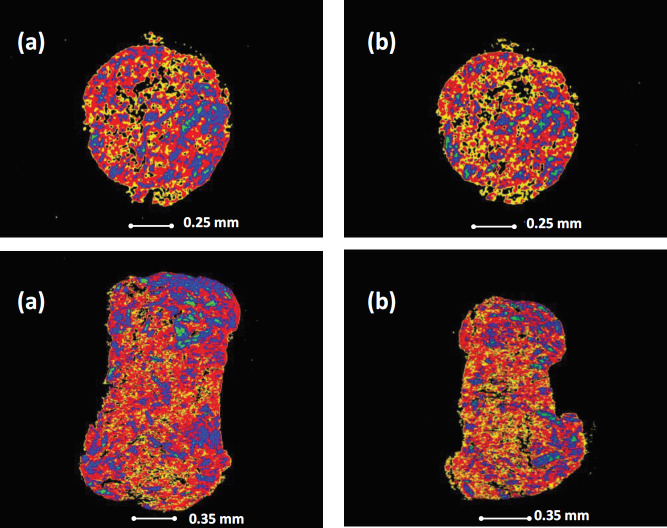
X-ray microtomographic sagittal and diametric images of Liqui-Pellet formulations of (a) F6 (b), and F7 detailing the homogeneity of the formulation mix of the Liqui-Pellet technology. Please see Table 1 for the composition of F6 and F7
